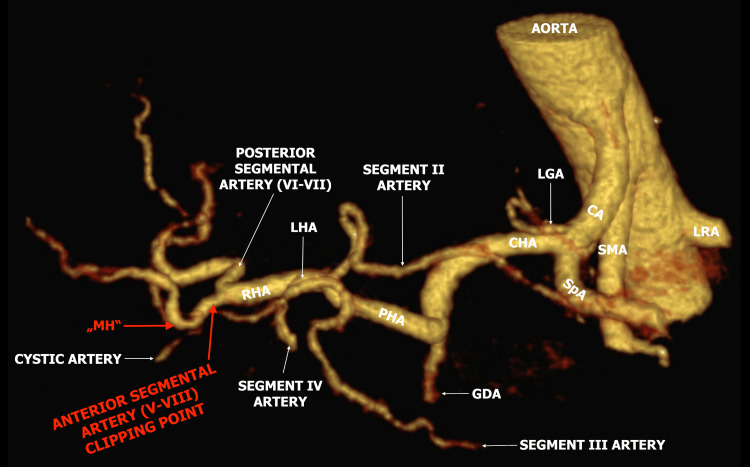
Older preoperative CT angiography scan MH: Moynihan’s hump; RHA: Right hepatic artery; LHA: Left hepatic artery; GDA: Gastroduodenal artery; CHA: Common hepatic artery; LGA: Left gastric artery; SpA: Splenic artery; CA: Celiac artery; SMA: Superior mesenteric artery; LRA: Left renal artery. (Courtesy of PD Jonas Ströder.)
